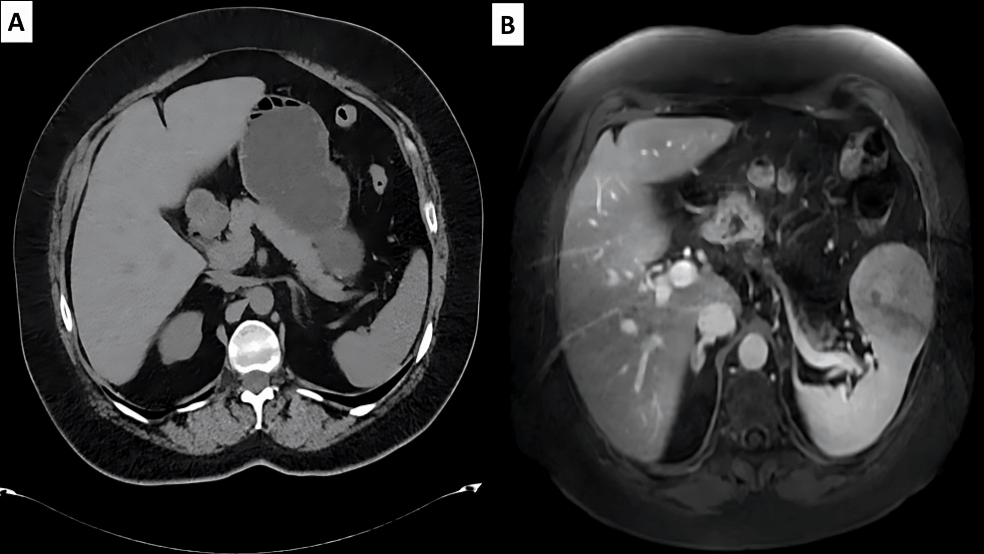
Computed tomography scan and magnetic resonance imaging at the splenic level (A) Computed tomography scan in 2012 in which a spleen of normal morphology and size was identified. (B) Magnetic resonance imaging in 2019 showing a well-circumscribed solid splenic lesion dependent on its anterolateral border of approximately 6.7 x 6.6 cm; the lesion is predominantly hypointense on T2 sequence with areas of central necrosis.
